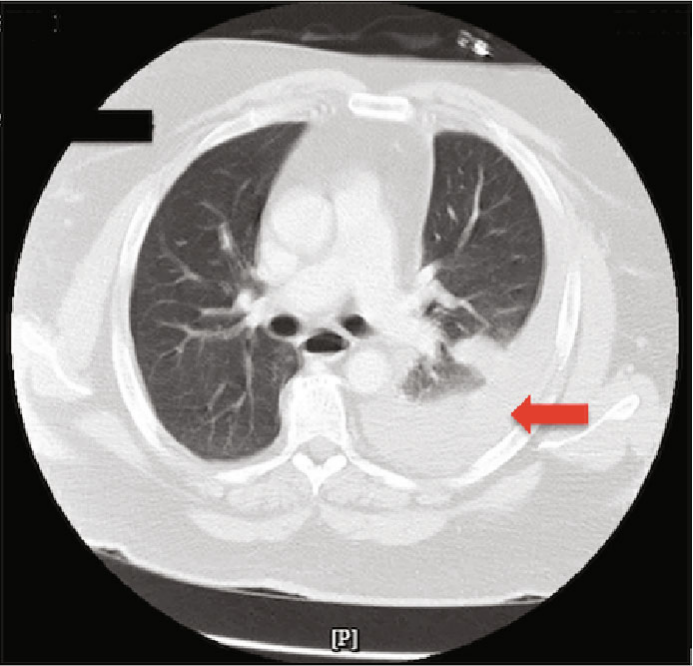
CT of the chest axial view done on Day 5 shows a large left pleural effusion (red arrow) with no contusion observed.
